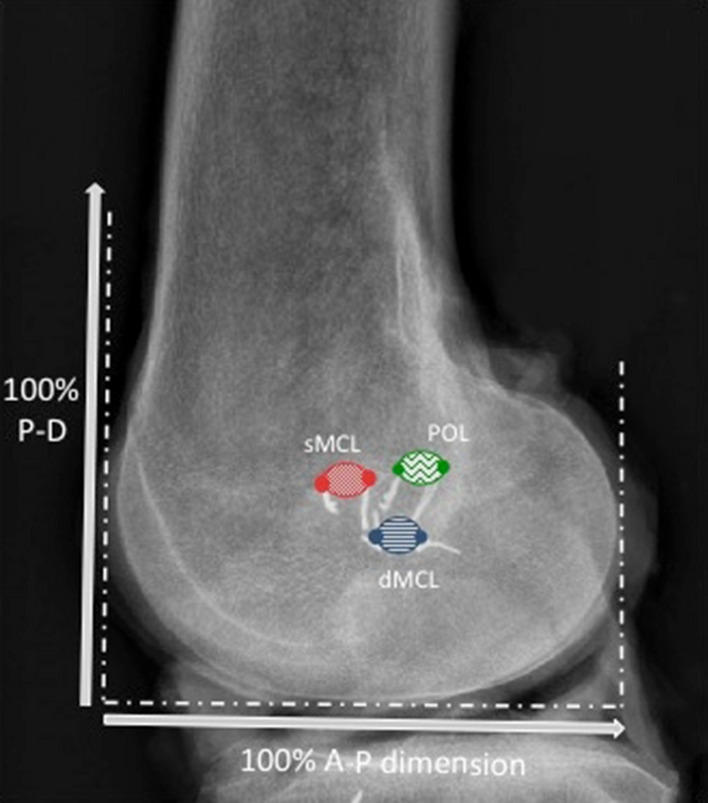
Femoral attachments of the superficial MCL (sMCL), deep MCL (dMCL) and the posterior oblique ligament (POL) as seen radiographically, in relation to the normalisation as a percentage of the AP size of the medial femoral condyle. Note that the wire staples were inserted into the bone with the fold in the staple at the point of interest, and the radiographic study required identification of which end of each staple was the correct measurement point at the surface of the bone (coloured areas). The P-D measurements use the same 100% normalising length as the A-P measurements
